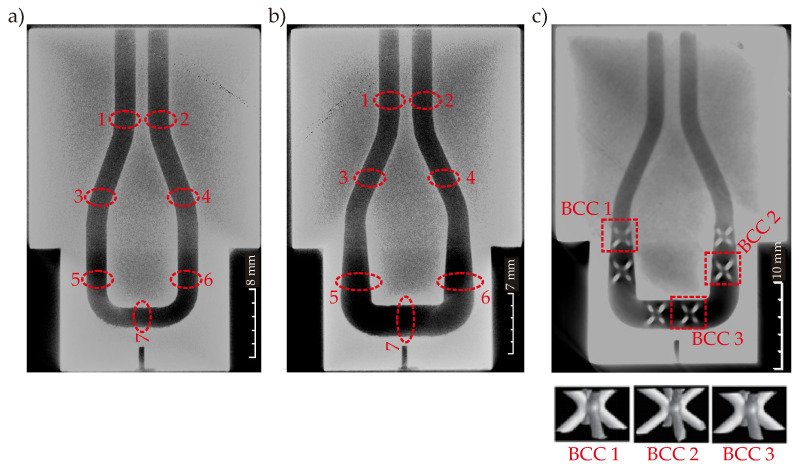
X-ray CT scan images of (a) circular CCC, (b) tapered CCC, and (c) tapered CCC with BCC lattice.
